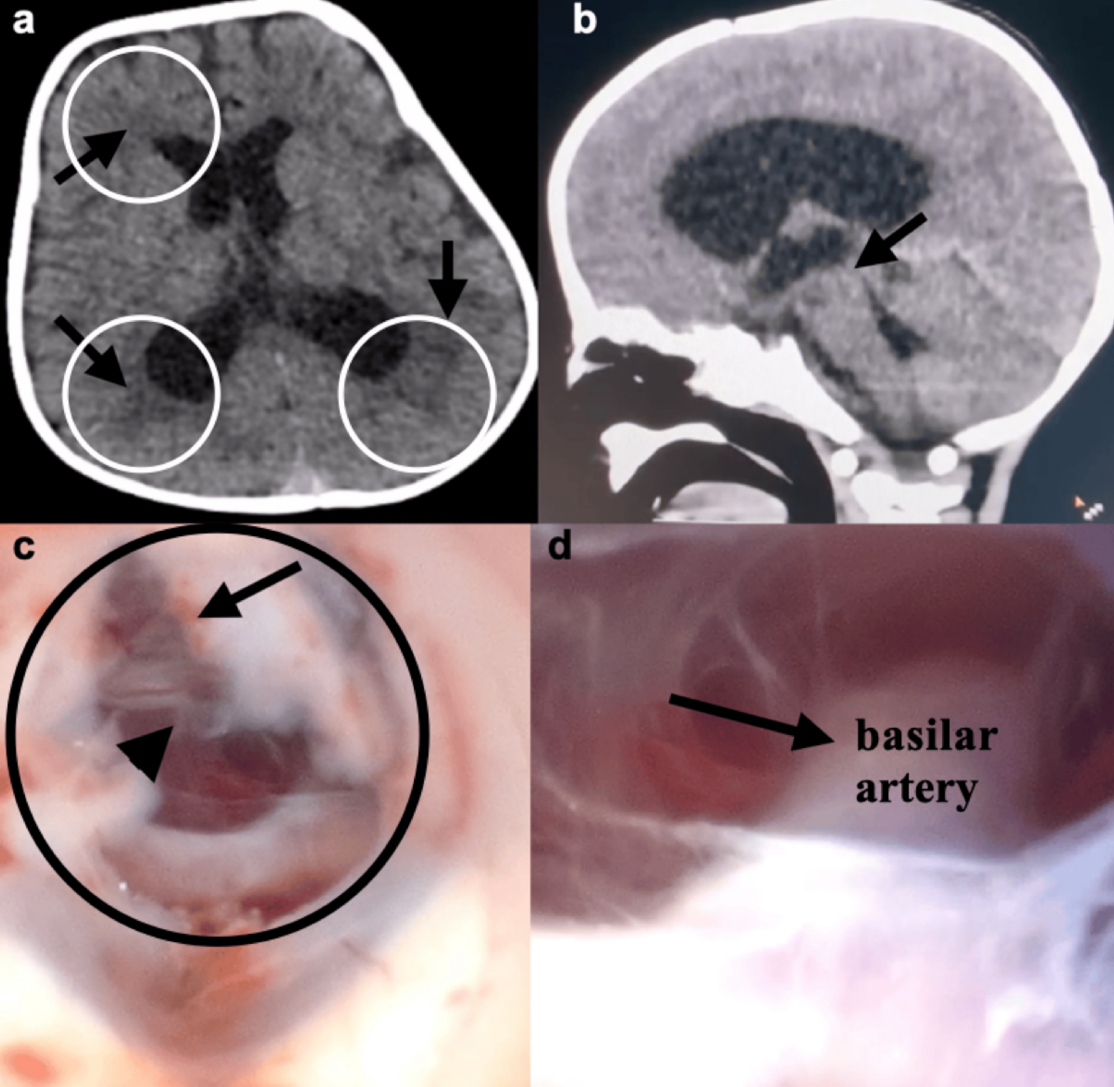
Computed tomography (CT) and endoscopic view (a) Axial CT scan of the skull showing hypertensive hydrocephalus with signs of ependymal transudation (black arrows). (b) Sagittal CT scan of the skull showing dilatation of the lateral ventricles and third ventricle with possible stenosis of the aqueduct of Sylvius (black arrow). (c) In the endoscopic view, it shows the tuber cinereum (black circle) with the first (black arrow) and second (black arrowhead) Liliequist membrane. (d) In the endoscopic view showing the prepontine cistern with the basilar artery (black arrow).
